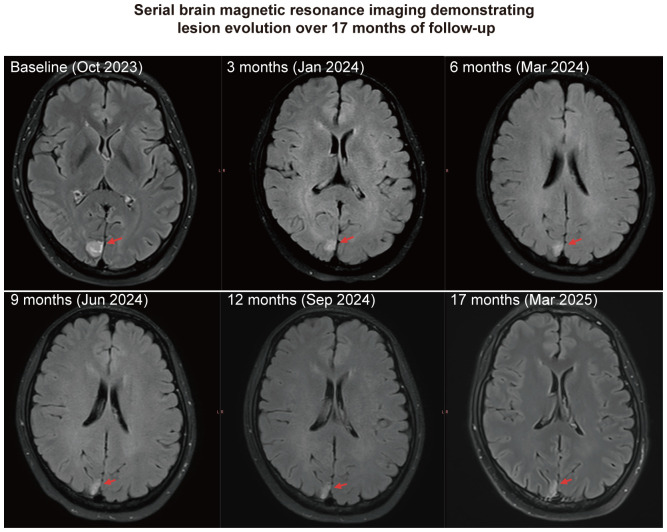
Serial brain magnetic resonance imaging demonstrating lesion evolution over 17 months. Representative axial T1-weighted contrast-enhanced brain MRI sequences from baseline (October 2023) to 17-month follow-up (March 2025). Red arrows indicate the dominant target lesion. Images demonstrate sustained intracranial disease control.
